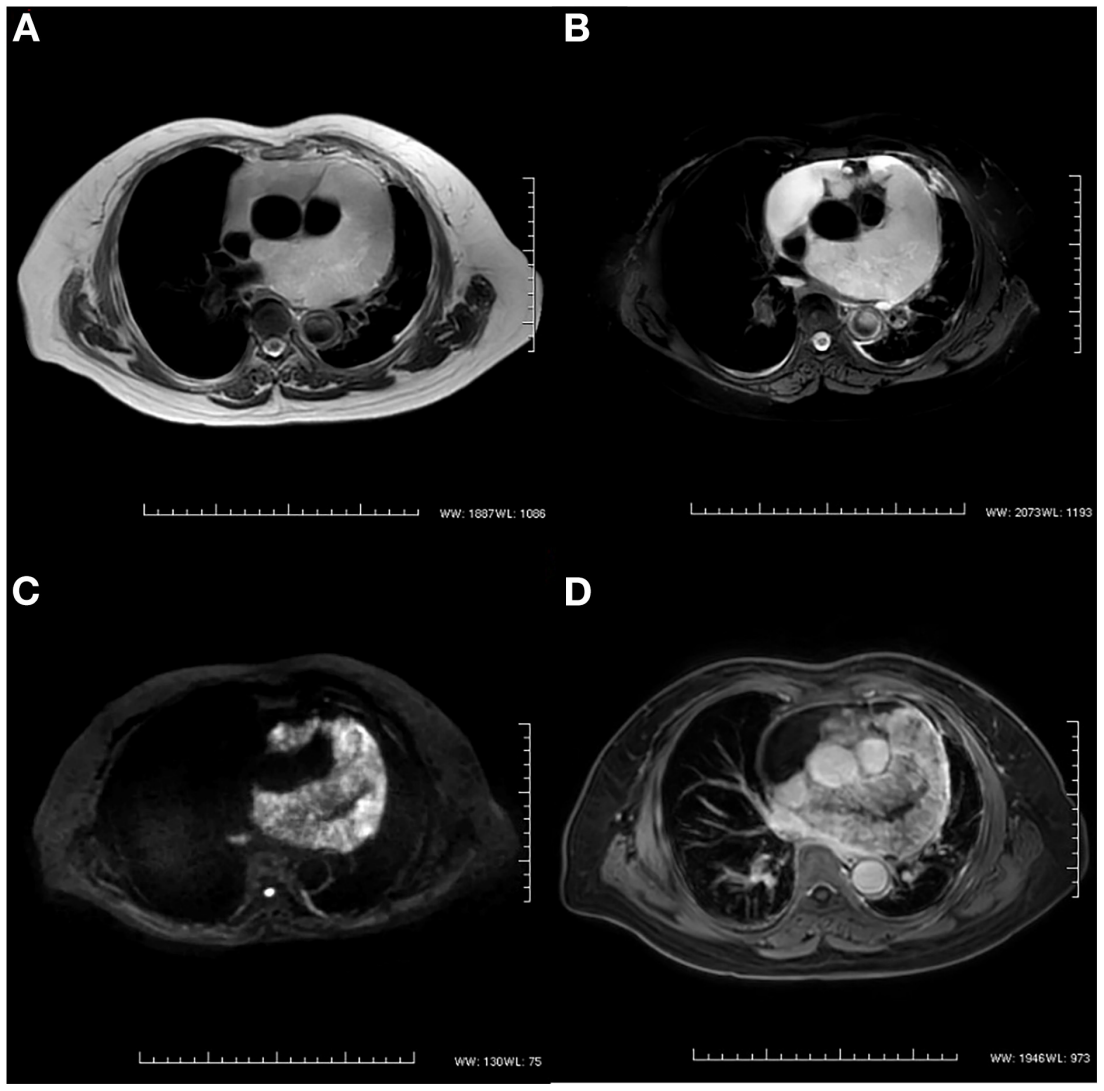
Cardiac magnetic resonance imaging. (A) T2-weighted imaging showed an irregular mass in the pericardium. (B) The signal intensity of the mass was hyperintense on T2-weighted imaging with fat suppression. (C) The diffusion-weighted image was hyperintense. (D) The mass showed irregular enhancement with gadolinium injection on T1-weighted imaging.
